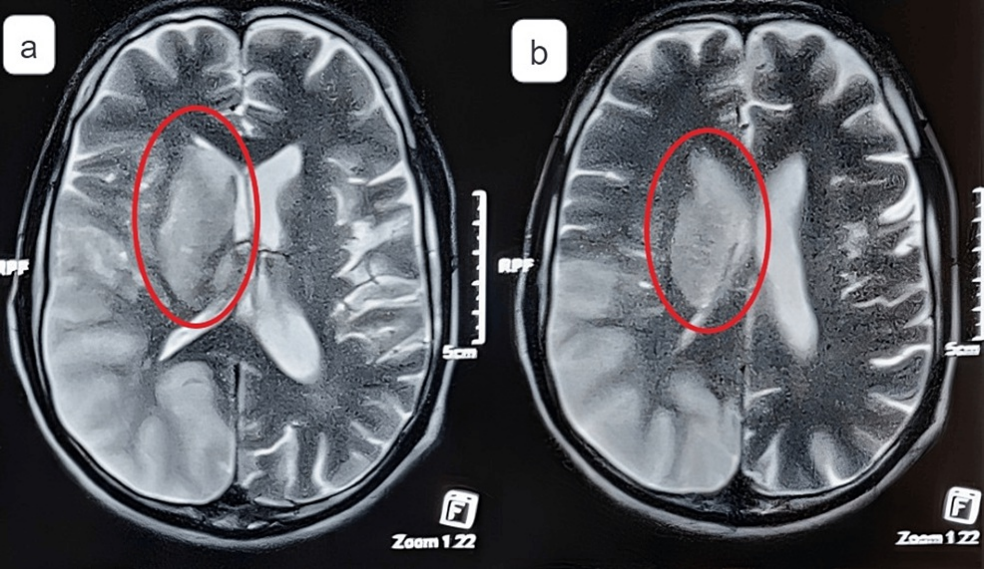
MRI reveals an acute non-hemorrhagic infarct noted in the right parietal-temporal-occipital region, predominantly involving the cortex and right ganglio-capsular region. The right lateral ventricle was observed to have effaced due to the mass effect and midline shift of 4 mm toward the left side. A: acute non-hemorrhagic infarct involving the cortex and right ganglio-capsular region, B: acute non-hemorrhagic infarct involving the right ganglio-capsular region MRI: magnetic resonance imaging
